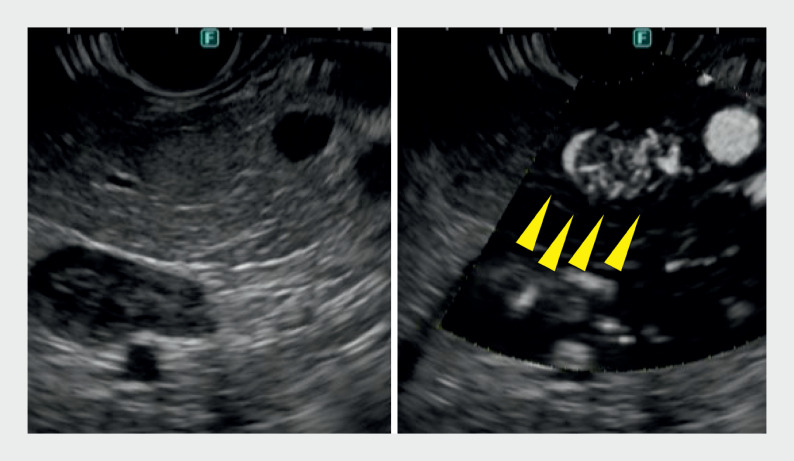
Detective flow imaging endoscopic ultrasound (DFI-EUS) detected a distinct hypervascular tumor (arrows). Strong blood flow effect was observed on the tumor surface and small vascular blood flow was observed in the interior.
